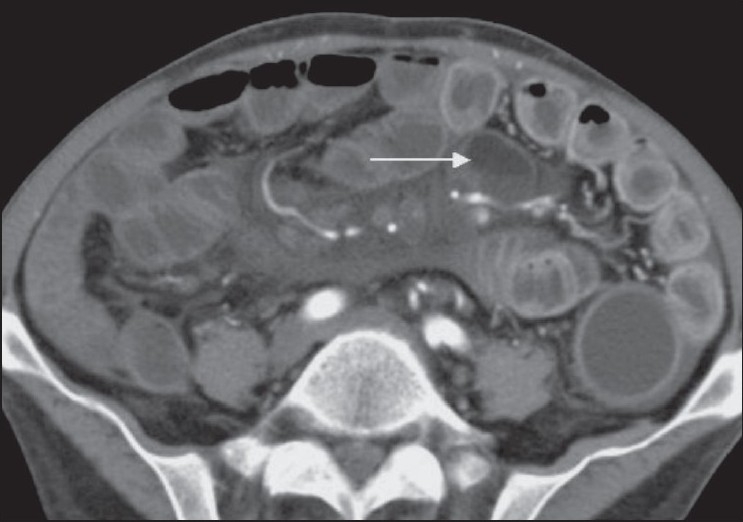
Axial CT scan shows mesenteric lymphadenopathy (arrow) with fat-fluid levels
